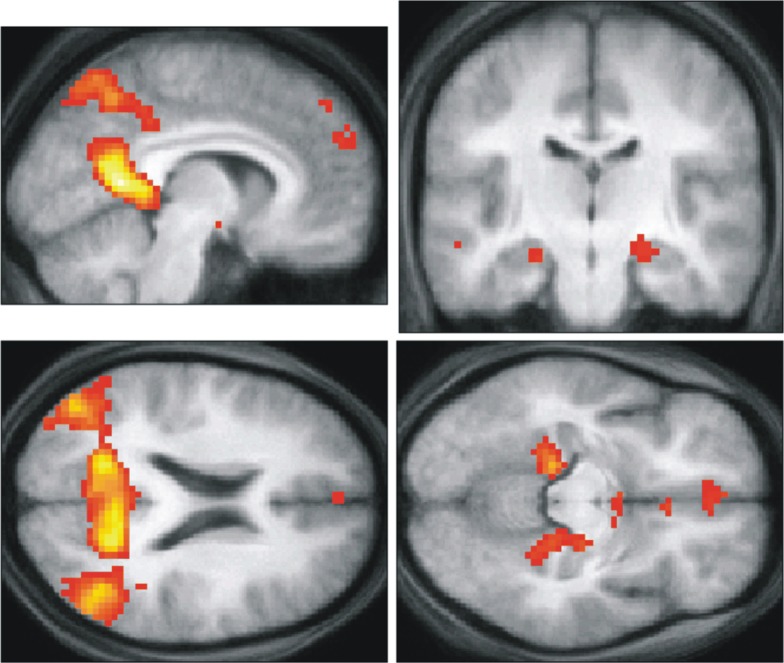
The “core network” for memory and imagination. These are the activations in common for recalling personal past events, recalling previously imagined scenes, and constructing novel scenes (from Hassabis and others 2007a). They are depicted on the averaged structural MRI brain scan of the study’s participants. Areas engaged, relative to baseline control tasks, included lateral and medial prefrontal cortices, precuneus, posterior cingulate, and retrosplenial cortices, lateral and medial temporal areas, including parahippocampal cortex and the hippocampus.
